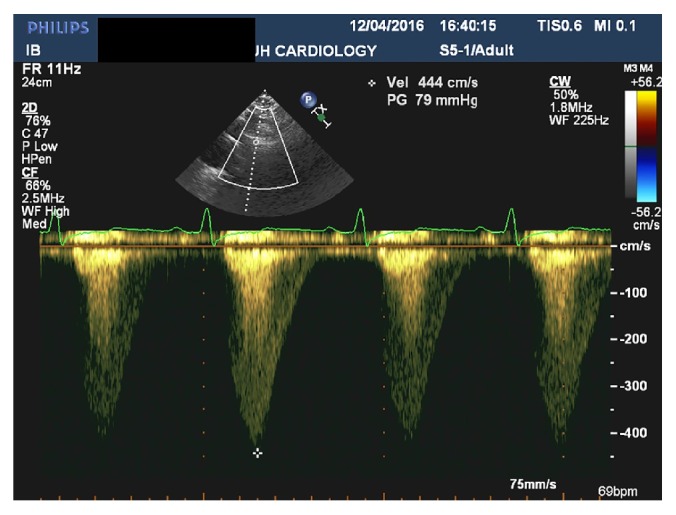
Suprasternal view of TTE with Doppler showing a peak gradient of 79 mmHg suggesting the presence of aortic coarctation.
